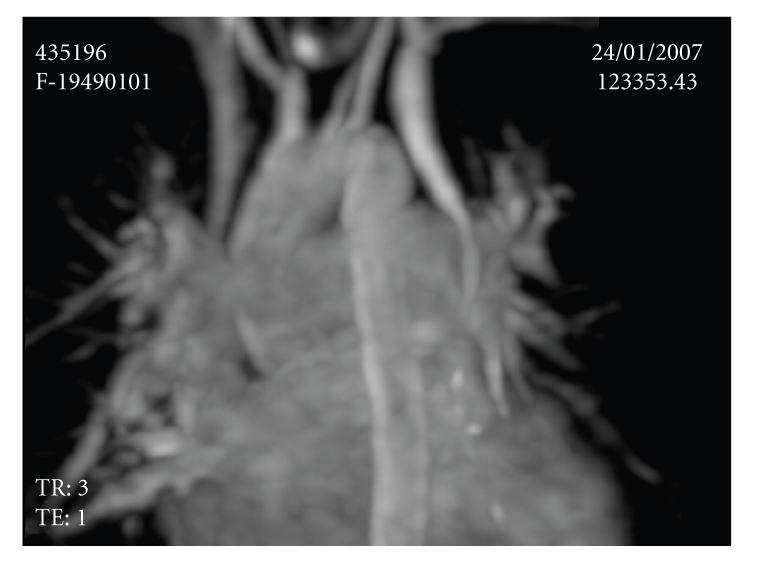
MRI showing 2 superior vena cavas, one on the right and the other on the left side.
